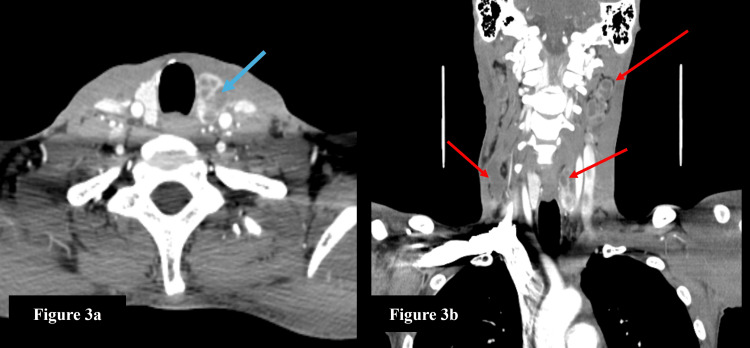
Contrast-enhanced CT of the neck a: The axial section of contrast-enhanced CT of the neck shows a well-defined multiloculated collection with enhancing septations within the left lobe of the thyroid gland (blue arrow); b: The coronal section of contrast-enhanced CT of the neck shows multiple, enlarged, and conglomerate cervical lymph nodes (red arrows) with non-enhancing hypodense necrotic areas on both sides.
